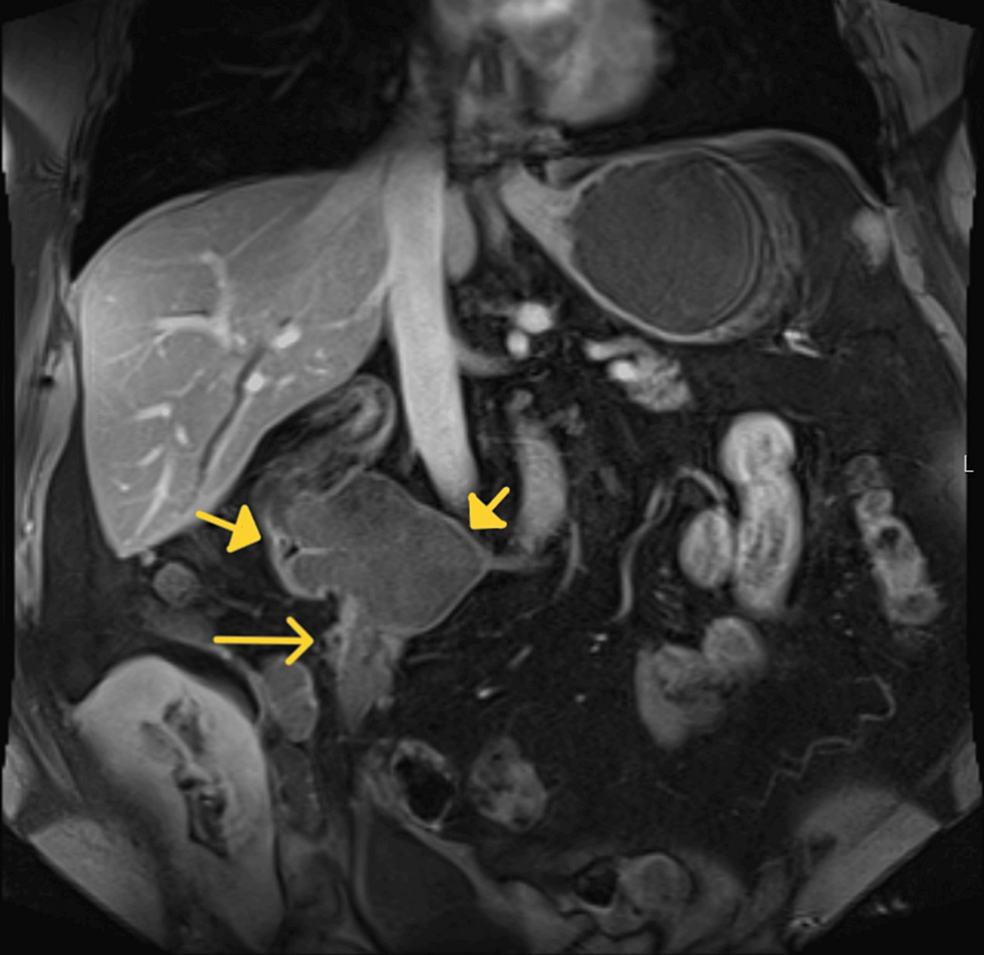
Coronal post-contrast fat-suppressed T1-weighted image shows a dilated right renal pelvis (solid arrows) and questionable linear enhancement along the lateral aspect of the right ureteropelvic junction (arrow)
